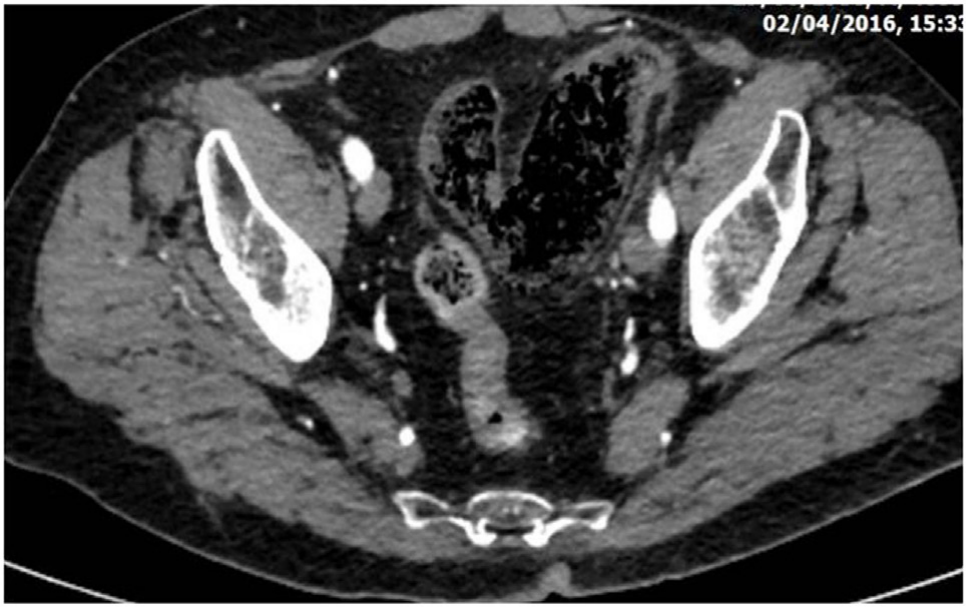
Abdominal CT revealing diffuse dilatation of the sigmoid with gas infiltration of its submucosa due to severe whole thickness ischemia (the gangrenous subtype).
